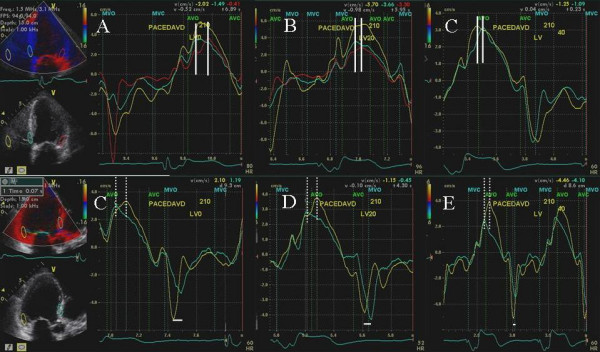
Figure shows tissue velocity maps obtained at baseline (A and C), during LV pre-excitation of 20 ms (B and D) and during LV pre-excitation of 40 ms (C and E) in apical 4 and 3 chamber views. Progressive decrease in septolateral wall delay (shown in B and C compared to A by white lines) and in septoposterior wall delay (shown in D and E compared to C by dotted white lines) occurred with progressively increasing LV pre-excitation. In addition progressive LV pre-excitation led to improvement in septoposterior wall delay in diastole (horizontal white lines, C-E).
